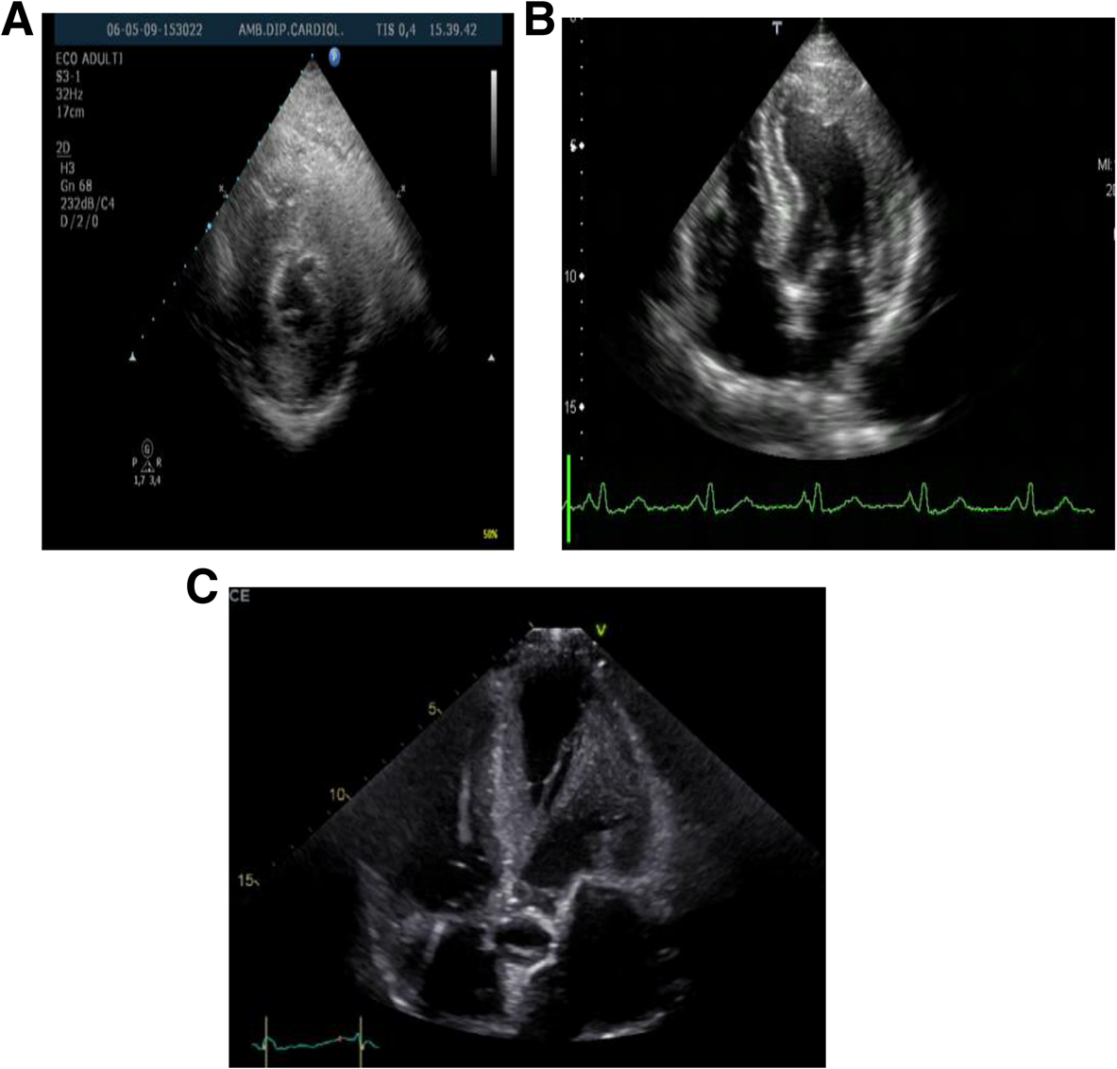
a, b, c Video. Short left echocardiogram (left) and 4-chamber apical (right) highlights the presence of concentric ventricular hypertrophy
